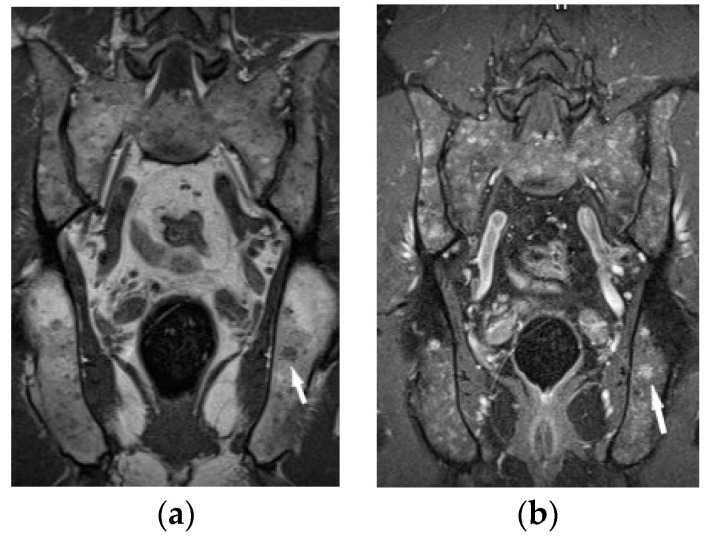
MRI of the pelvis. T1-weighted image, coronal plane (a), T1-weighed image with fat saturation and with contrast administration (b) shows multiple hypointense bone marrow lesions (a) with contrast enhancement ((b), white arrows).
